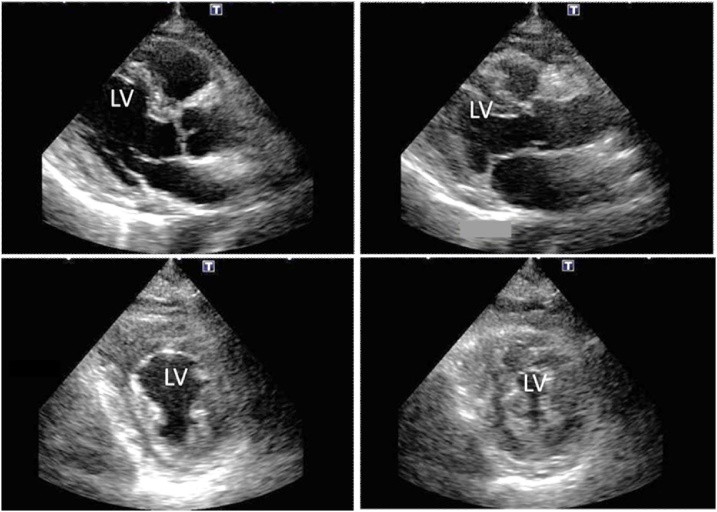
Echocardiogram on POD 44. Parasternal long axis views (top) and short axis views (bottom) of end-systolic frames (right) and end-diastolic frames (left) are shown. Normal left ventricular function with no wall motion abnormalities was found. LV; left ventricular.
